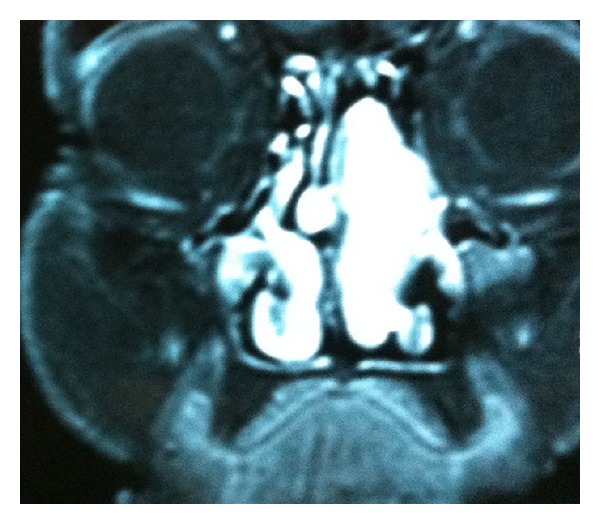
Coronal MRI view of glomangiopericytoma filling the left nasal cavity.
